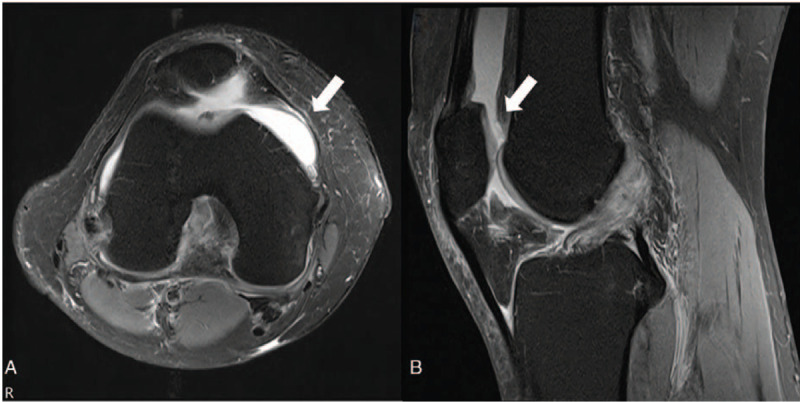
A) MRI knee Rt. (non-enhance sagittal view): immediately after injury. B) MRI knee Rt. (non-enhance axial view): joint fluid effusions.(arrow). MRI = magnetic resonance imaging.
